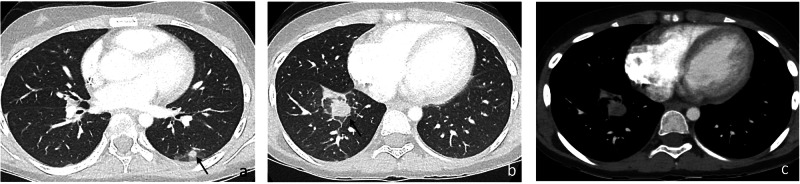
Initial invasive pulmonary aspergillosis in a 11-year-old girl with acute lymphoblastic leukemia (ALL) pre-B. Axial images of chest CE-CT scan in lung (a, b) and mediastinal (c) window show bilateral lung nodules with “halo sign” (arrows). Note the various size on the nodules and the central “hypodense sign” in the largest one (c)
